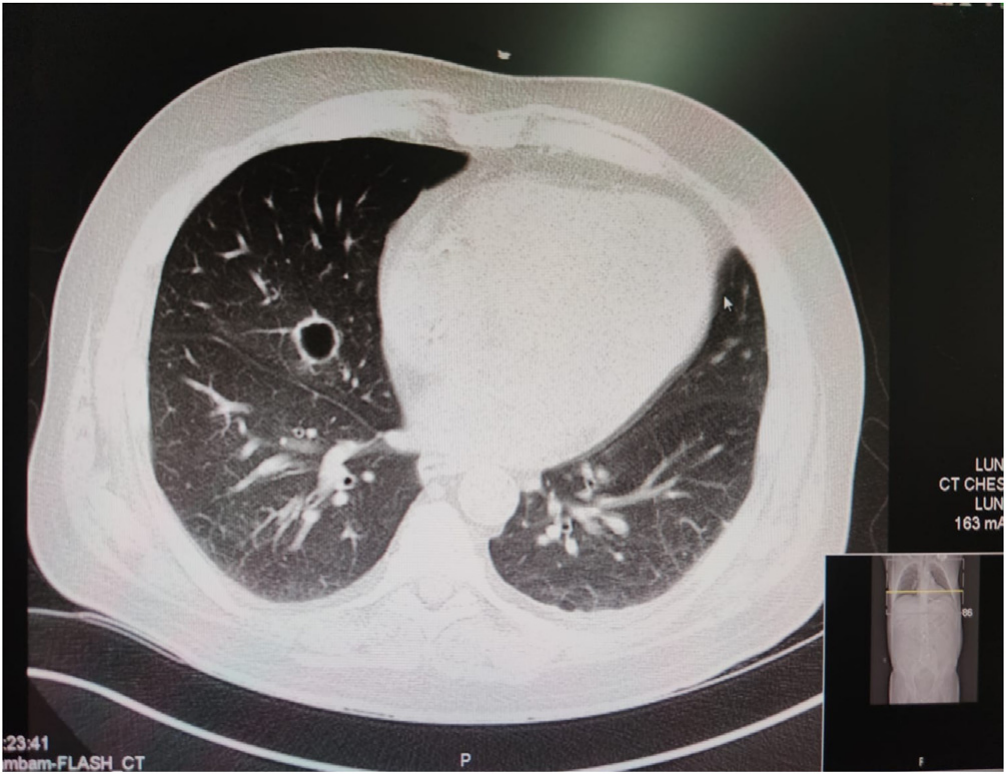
Thoracic CT scan bullous lesion suspicious for a cavitation in the right lower lobe with multiple acinar opacities.
